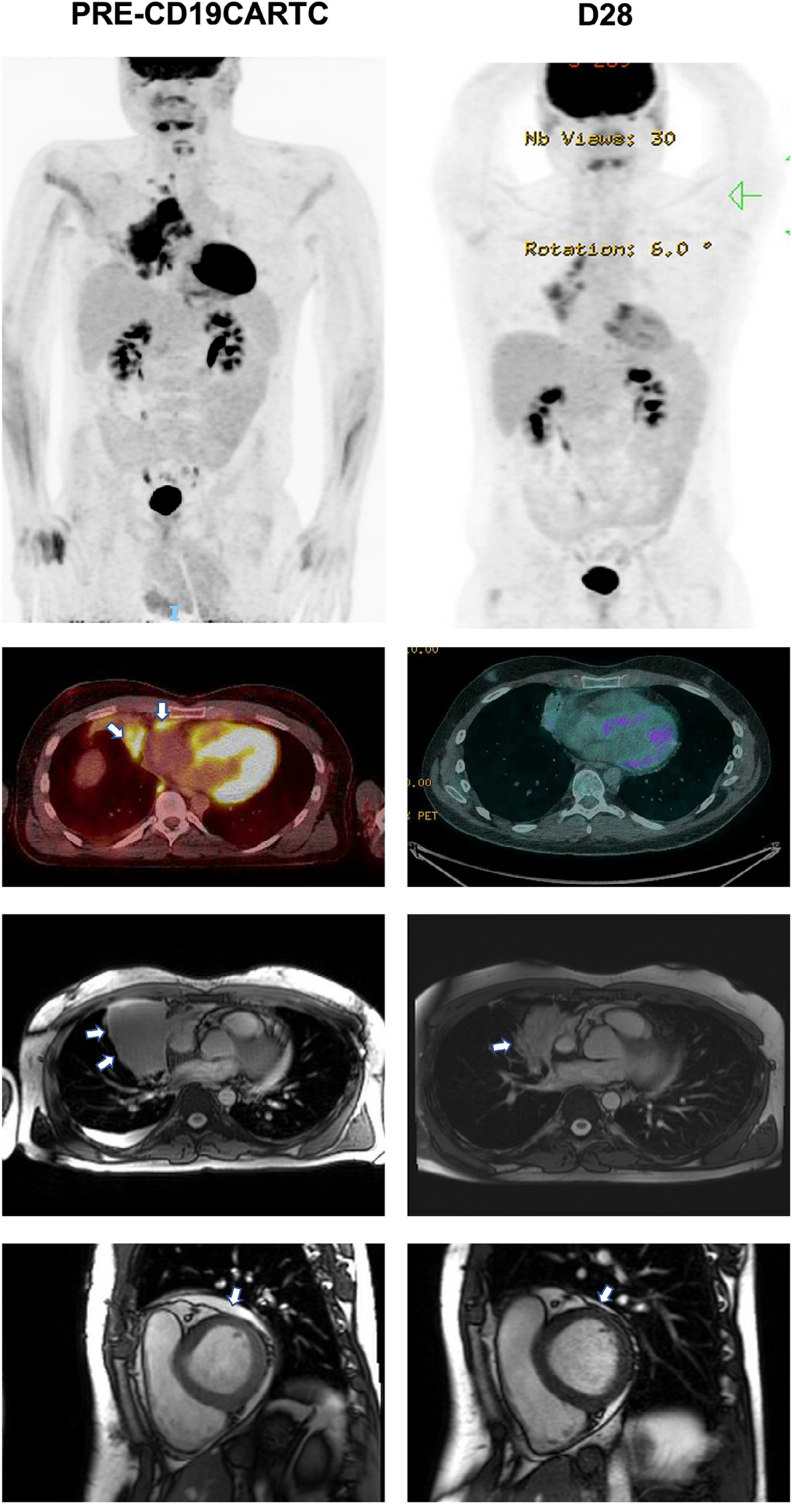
A 24‐year‐old male with relapsed/refractory (r/r) large‐B‐cell‐lymphoma (LBCL) was treated with CD19‐targeting chimeric antigen receptor T‐cell (CD19CAR‐T). Computed tomography‐positron emission tomography (CT‐PET) demonstrated a 77 × 58 mm mass at the right hilum adjacent to the right atrium, with disease extension into the pericardium which was FDG‐avid. Small pericardial effusion (8 mm) on cardiac magnetic resonance imaging (CMR); no endomyocardial tumor invasion. CMR at day 28 post‐CD19CAR‐T Reduction in the right hilar mass on CMR at day 28 post‐CD19CAR‐T and Deauville 4 response on PET‐CT.
